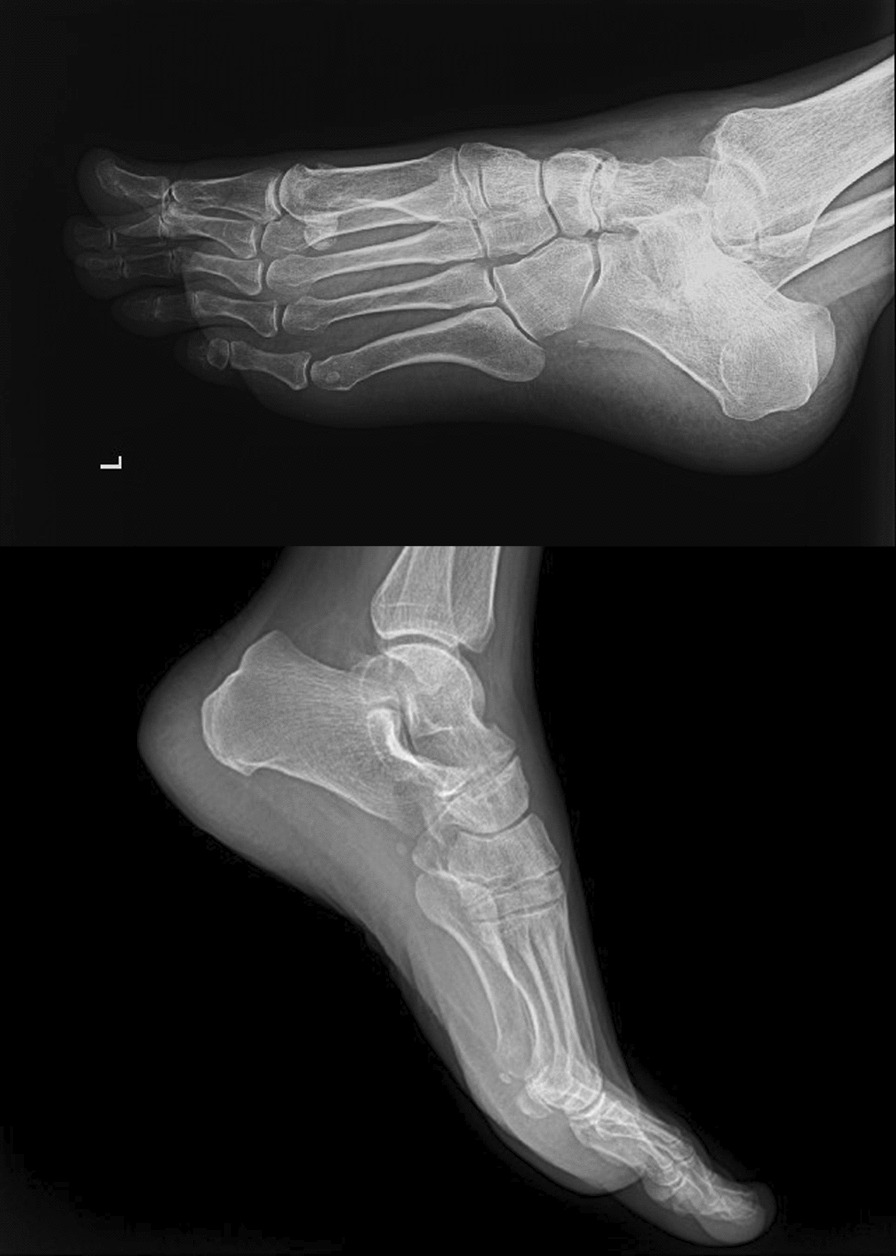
Oblique and lateral radiography showing cortical erosion and sclerosis and joint space narrowing in the talonavicular joint
